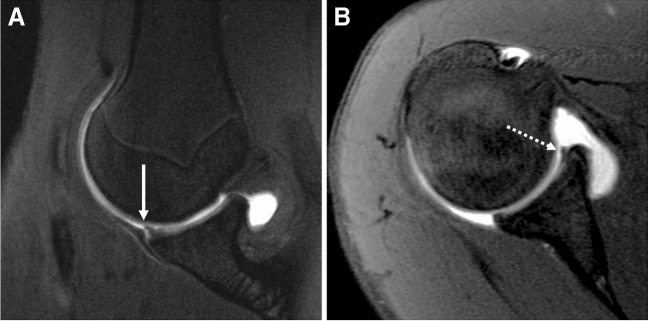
Abduction external rotation (ABER) positioning. Sixteen-year-old with history of recent shoulder dislocation. A T1-weighted fat-suppressed MR arthrogram image in the ABER position shows a tear of the anterior labrum which remains partially attached (arrow), consistent with a Perthes lesion. B On conventional axial T1-weighted fat-suppressed image, the tear is less evident (dashed arrow)
